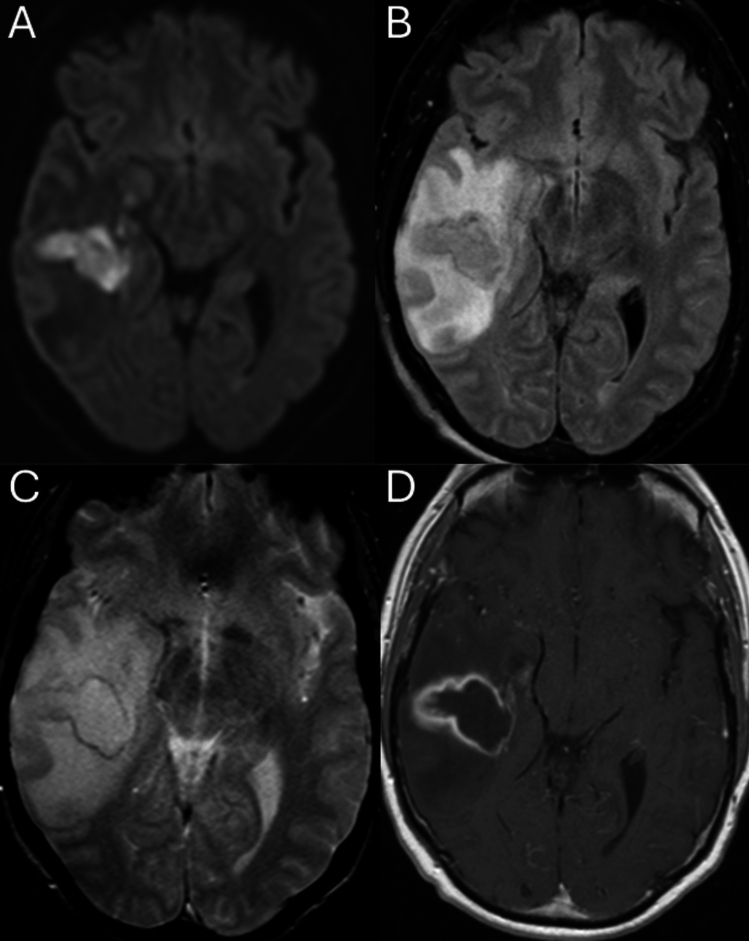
Magnetic resonance imaging of a patient with central nervous system nocardiosis. A Diffusion-weighted imaging shows marked internal diffusion restriction within the right temporal nocardia abscess. B FLAIR weighted images demonstrate marked perilesional edema. C Thin contiguous rim of hypointensity on gradient-recall echo. D Thick-walled peripheral enhancement of the abscess on postcontrast T1-weighted imaging, characteristic of a late capsule pyogenic abscess
